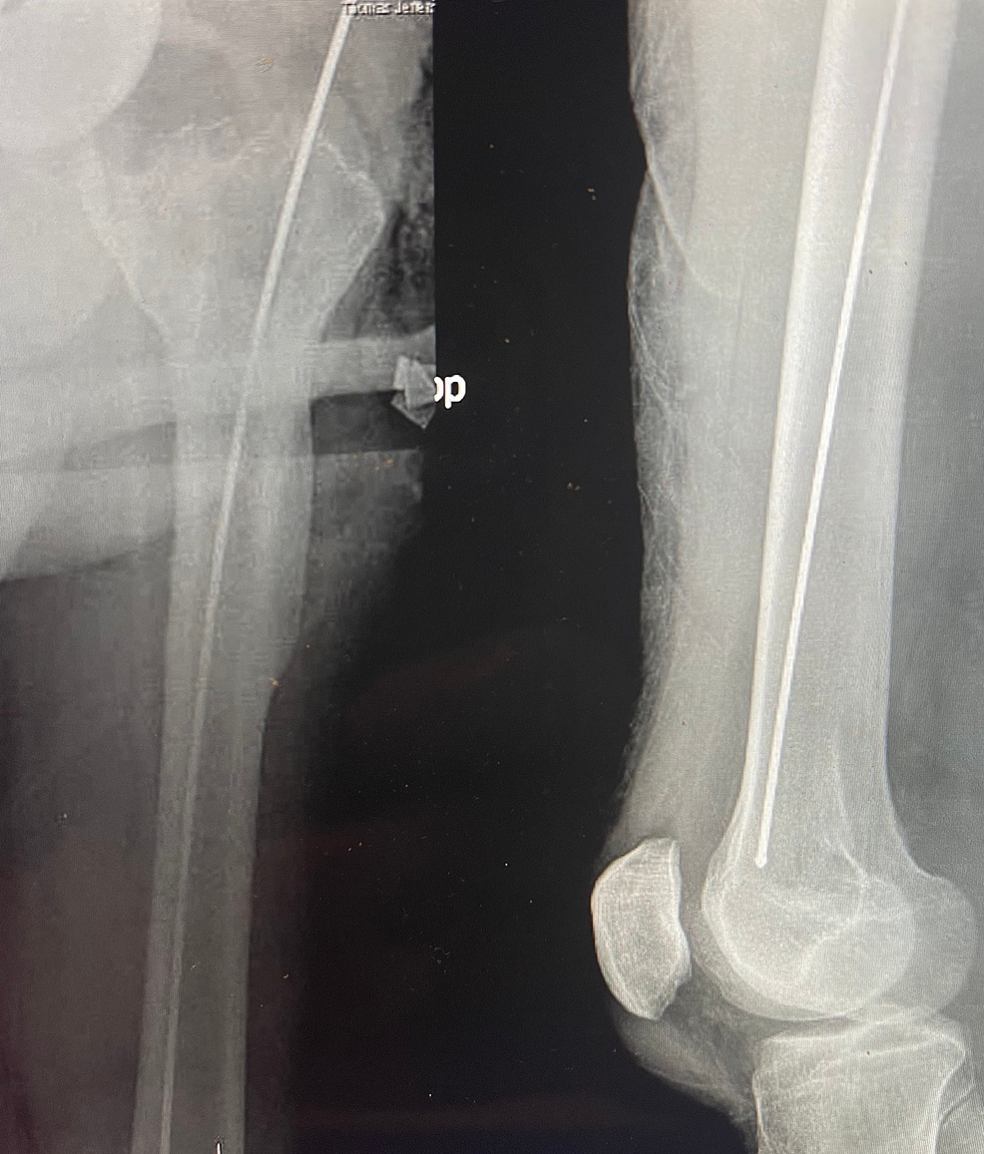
Intraoperative radiographic images demonstrating intramedullary positioning of a ball-tipped guide wire
